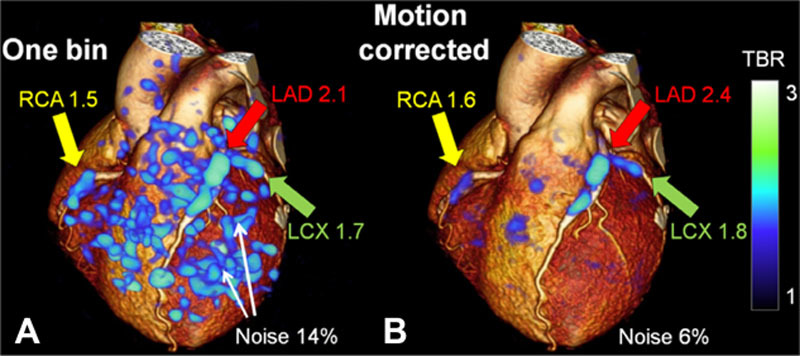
Cardiac motion-corrected ^18^F-NaF positron emission tomography (PET). 3D cardiac computed tomographic (CT) rendering with superimposed ^18^F-NaF cardiac-gated PET image reconstruction using a single bin (25% of PET counts) vs (B) motion corrected PET with 10-gated bin method (consecutive 10% segments), resulting in less noise and improved target to background ratio. Adapted from Rubeaux et al^208^ with permission of the publisher. Copyright ©2016, Society of Nuclear Medicine and Molecular Imaging, Inc. LAD indicates left anterior descending artery; LCx, left circumflex artery; and RCA, right coronary artery.
